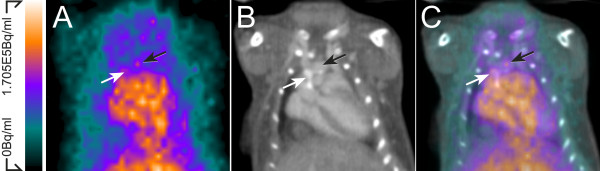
In vivo *imaging. (A) *In vivo *PET, (B) CT angiography, and (C) fused PET/CT images demonstrate the high ^68^Ga signal in the blood pool as seen in the aorta and heart. Calcified (white arrows) and non-calcified (black arrows), aortic arch and aortic root can be seen in contrast-enhanced CT angiography.
